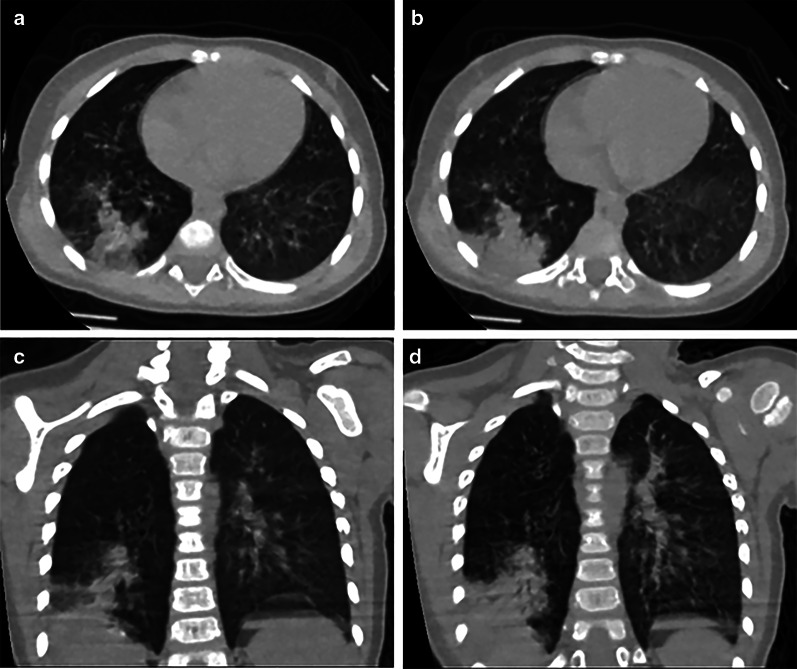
A two-year-old male child with positive RT-PCR findings for COVID-19. a–d Noncontrast axial CT images of the chest show right lower-lobe peripheral consolidation and surrounding ground-glass opacity. This is in keeping with his CO-RADS 4 and indeterminate RSNA classification
